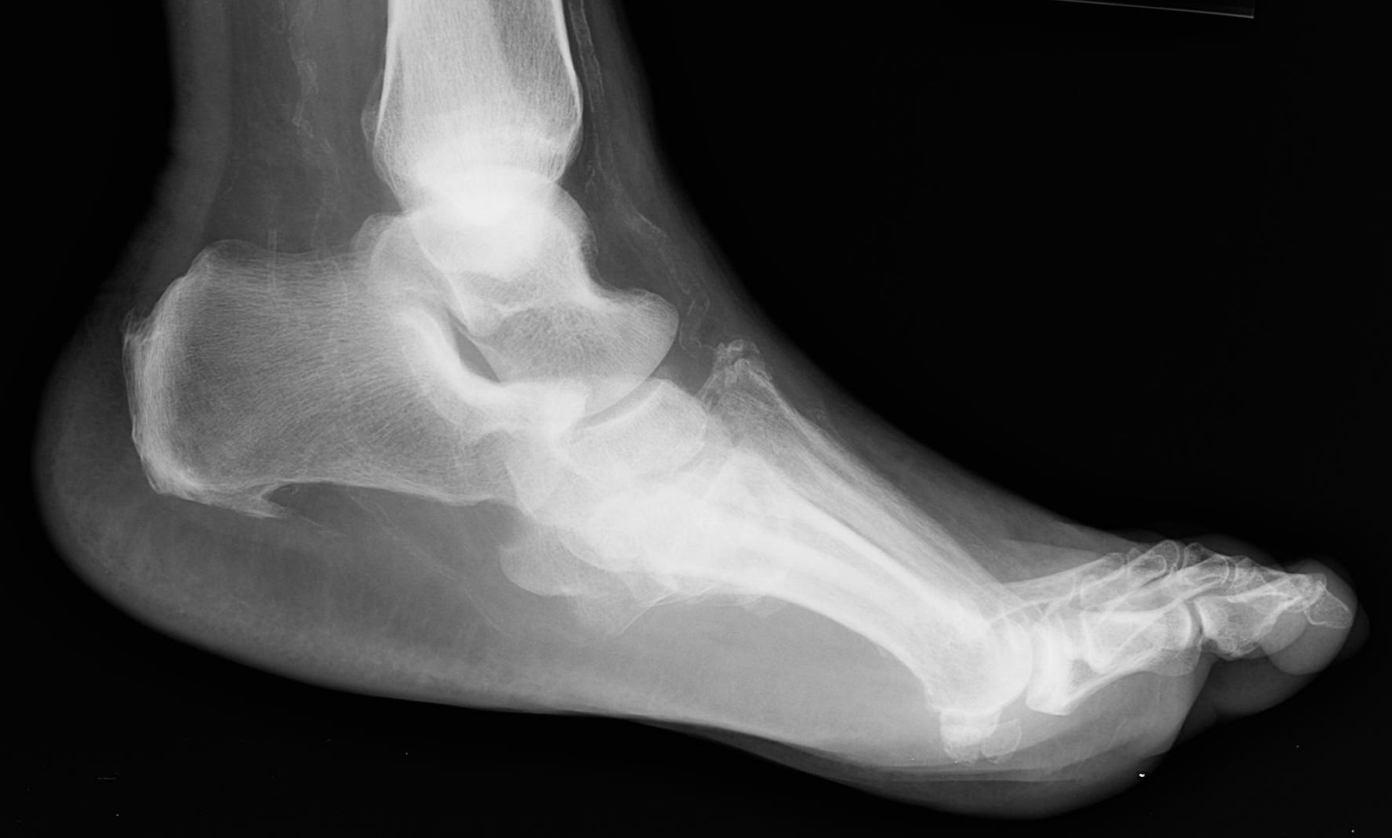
Lateral radiographic view showing the severe midfoot fracture-dislocation of the diabetic CN foot.
